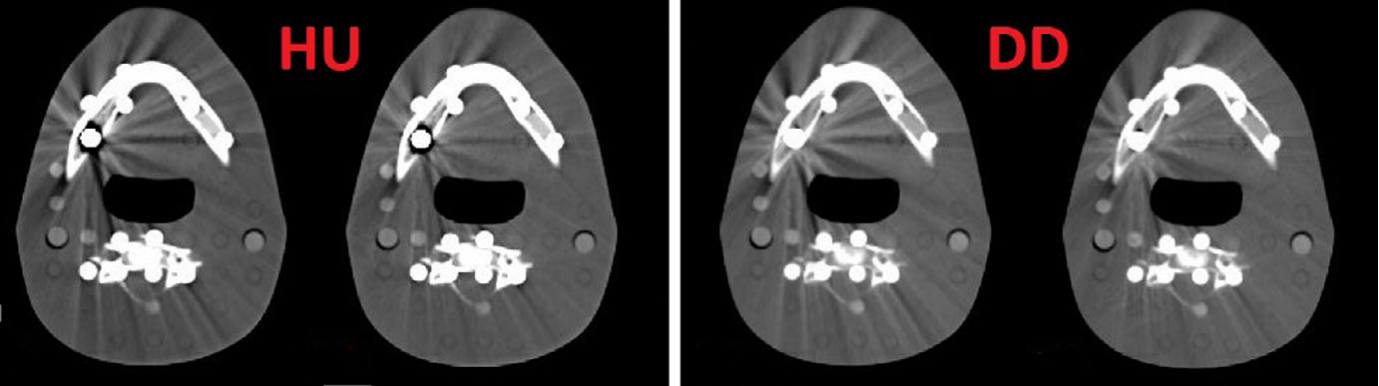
CT images with brass inserts (left on both panels) and the iMAR algorithm (right on both panels) for the HU reconstruction (left panel) and the 120 kVp DD reconstruction (right panel).
